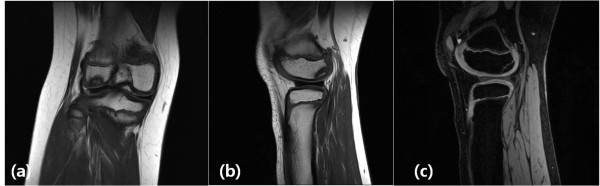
(A, B) MRI study showing a discoid lateral meniscus with a 1.5 by 1.5 cm osteochondral lesion involving the posterior articular surface of the lateral femoral condyle. (C) There was no evidence of fluid signal intensity between host and fragment on a T2-weighted MRI scan.
